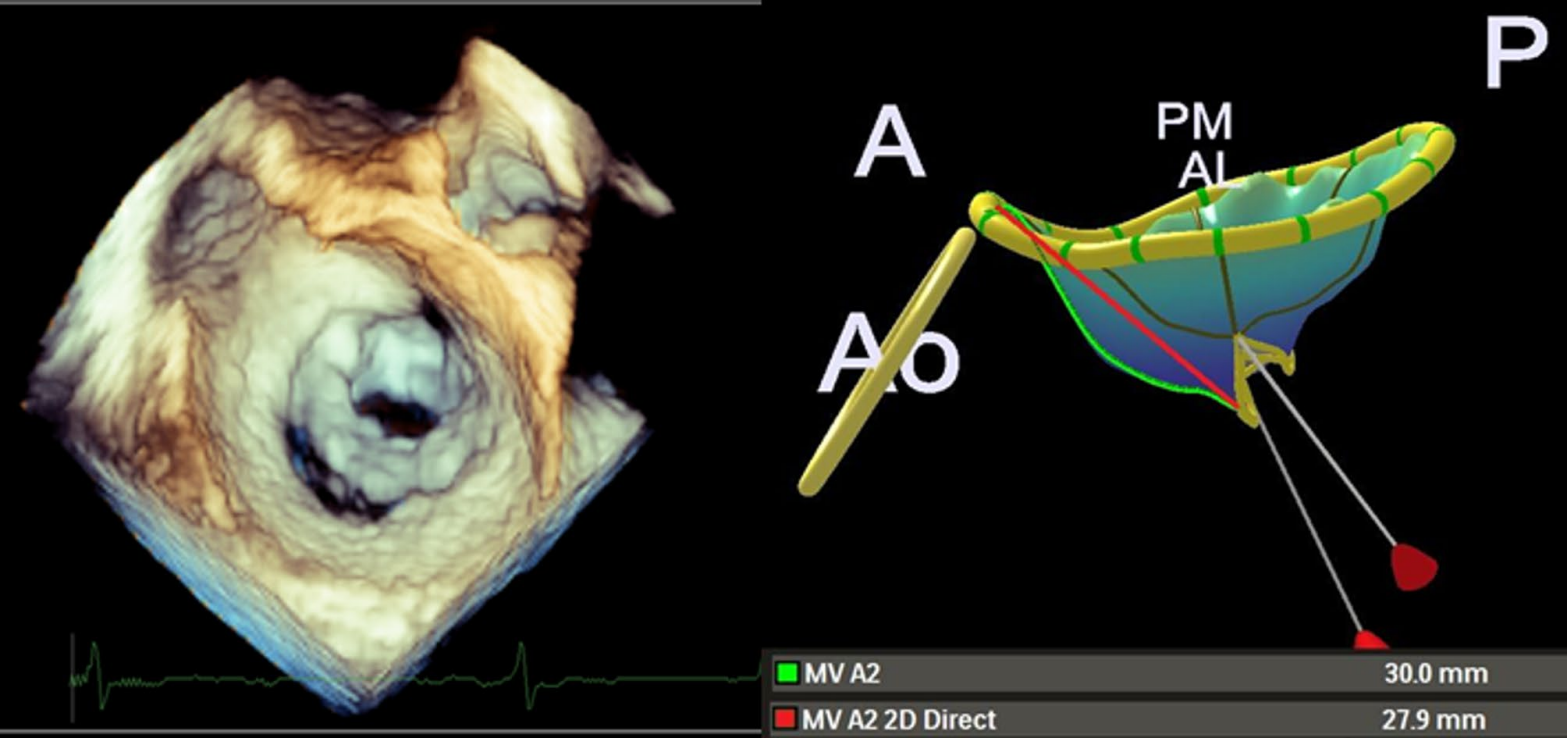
Three-dimensional transoesophageal echocardiography (3DTOE) in zoom mode demonstrating severe mitral stenosis with commissural fusion (left) and the corresponding reconstructed mitral valve model (right)
